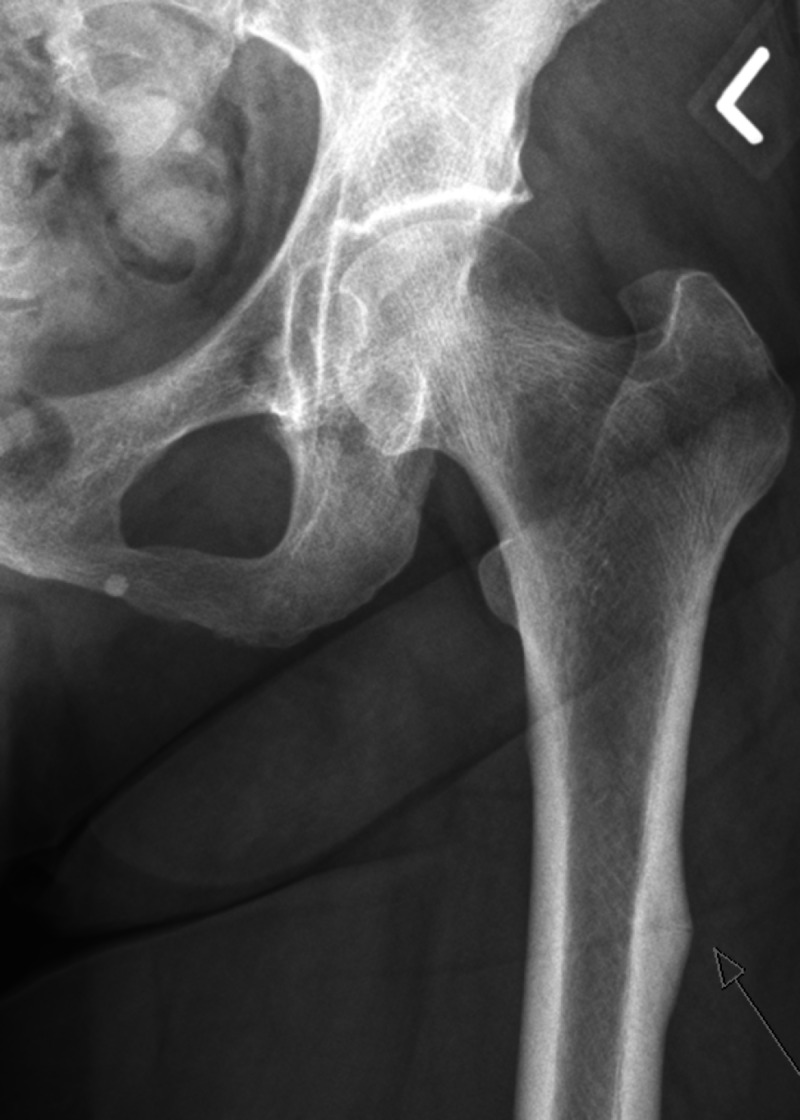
X-ray left hip shows a left atypical femoral fracture (AFF) in the proximal femur The cortical periosteal thickening is adjacent to the faint translucent fracture that is extending from the lateral cortex into the medulla.
